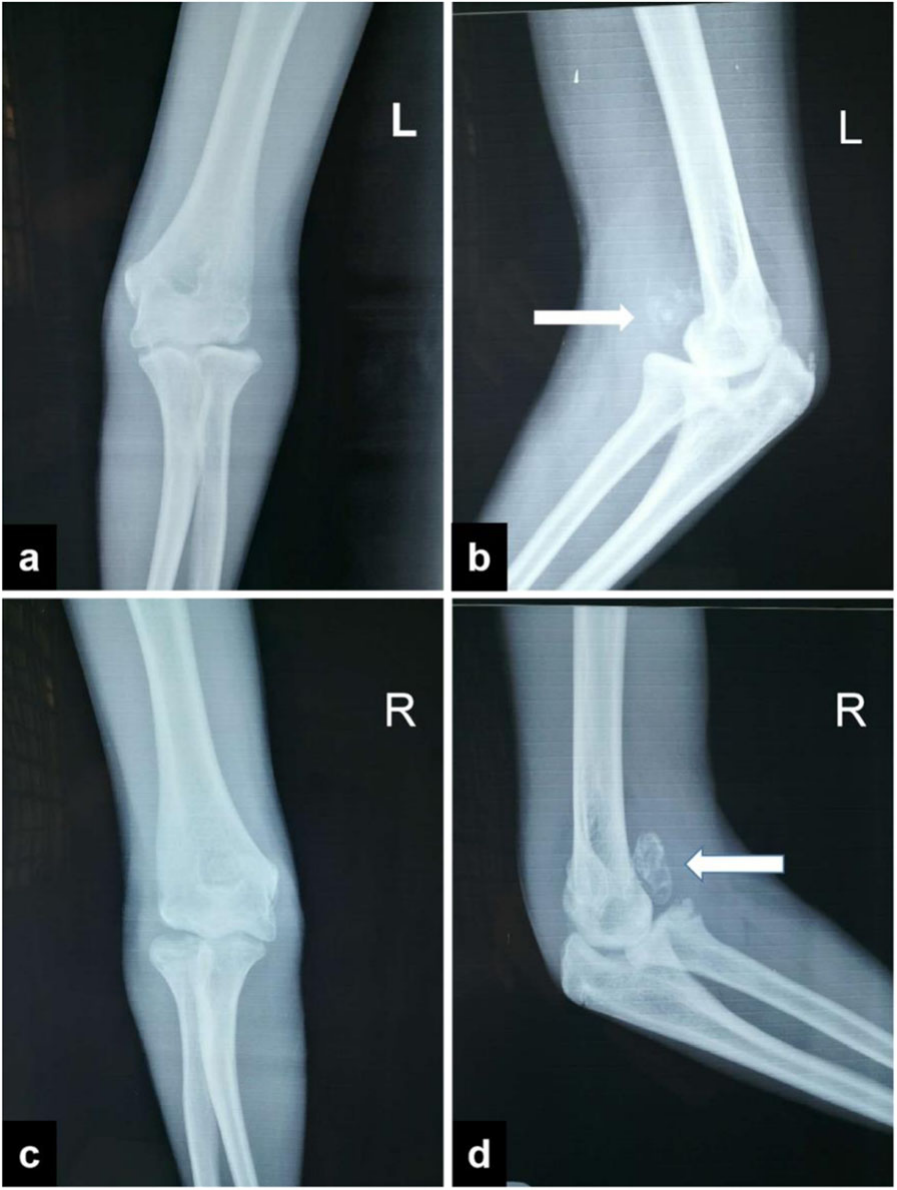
The initial X-ray (a, c, anteroposterior; b, d, lateral) of bilateral elbows showed calcified densities surrounding anterior and posterior aspects of the elbow joints. (b, d (arrow))
